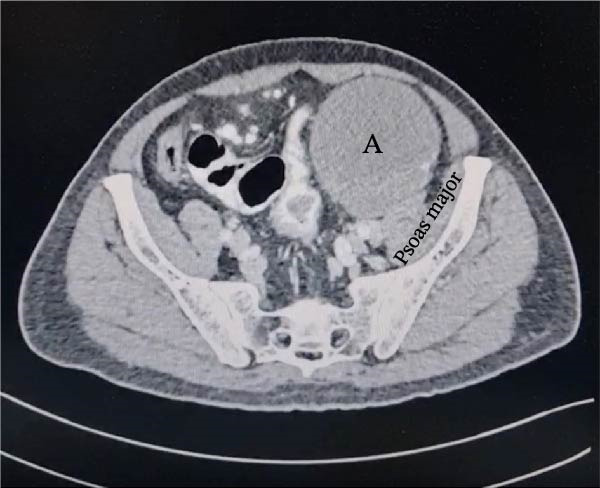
Axial CT scan showing hypodense left pelvic mass (A) measuring 9.0 cm × 7.9 cm × 9.5 cm.
